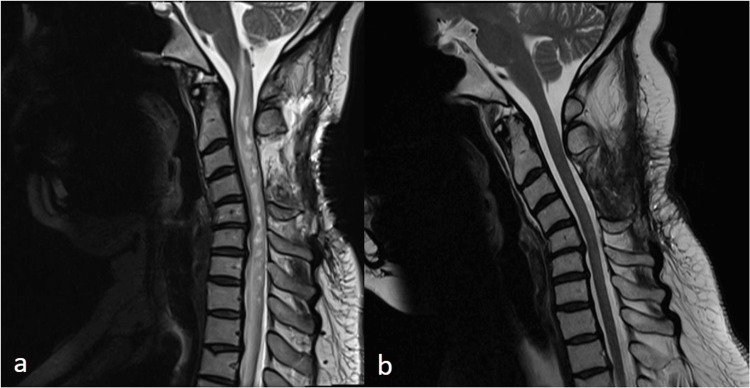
Sagittal T2 weighted magnetic resonance images of the cervical spine at one month post-operatively (a) and six months post-operatively (b). Images at one month demonstrate worsening enhancement within the dorsal cord, new enhancement within the operating site and cervical kyphosis. At six months there is no residual abnormal signal or pathologic contrast enhancement within the dorsal cord to suggest progressive or recurrent spinal cord disease.
